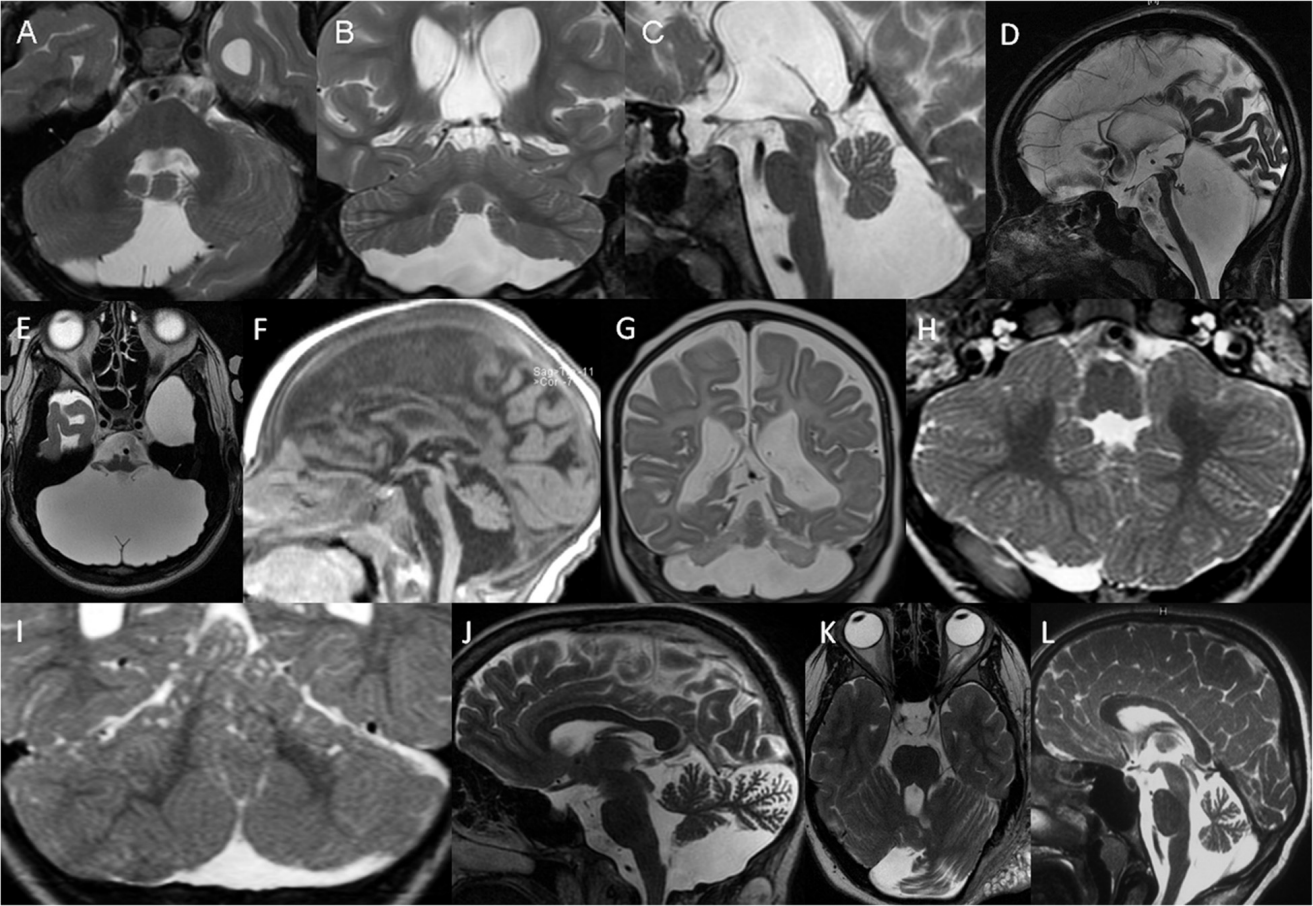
a, Axial, b, Coronal, and c, Sagittal T2-weighted images of a 17-year-old male with seizures and cerebellar hypoplasia of unknown origin show reduced volume, but near normal structure of the cerebellum. In addition, a cavum Vergae and reduced periventricular bilateral white matter are noted. d Sagittal and e, Axial T2-weighted images of a 15-year-old girl with cerebellar agenesis reveal almost complete absence of cerebellar tissue except for a rudimentary structure projecting posterior to the inferior colliculi and lateral to the brainstem. The pons is markedly hypoplastic and the posterior fossa is enlarged (reprinted with permission from Poretti A et al., Eur J Paediatric Neurol, 2009). f Sagittal T1- and g Axial T2-weighted images of a 1-year-old child with pontocerebellar hypoplasia type 2 and *TSEN54* mutations show marked hypoplasia and reduction in the size of the cerebellar hemispheres with relative preservation of the midline vermis, resulting in a characteristic “dragonfly” appearance (reprinted with permission from Bosemani T et al., Radiographics, 2015). h Axial and i, Coronal T2-weighted images of an 11-month-old child with Poretti-Boltshauser syndrome and *LAMA1* mutations reveal bilateral cerebellar dysplasia as abnormal cerebellar foliation, white matter arborization, and gray-white matter junction and multiple cortical/subcortical cysts located within the cerebellar vermis (mostly anterior and superior part) and both cerebellar hemispheres (mostly posterior and superior parts) (reprinted with permission from Poretti A et al., Cerebellum, 2014). j Sagittal and k, Axial T2-weighted images of 15-year-old patient with neurofibromatosis type 1 and cerebellar dysmorphia show enlargement of the left cerebellar hemisphere with enlarged interfolial spaces and bulky appearance of its posteromedial part, which crosses the midline. In addition, a plexiform neurofibroma is seen in the left soft tissue (reprinted with permission from Toelle SP et al., Cerebellum, 2015). l Sagittal T2-weighted image of a 5-year-old child with ataxia oculomotor apraxia type 1 disease and marked cerebellar atrophy as enlargement of the interfolial spaces
